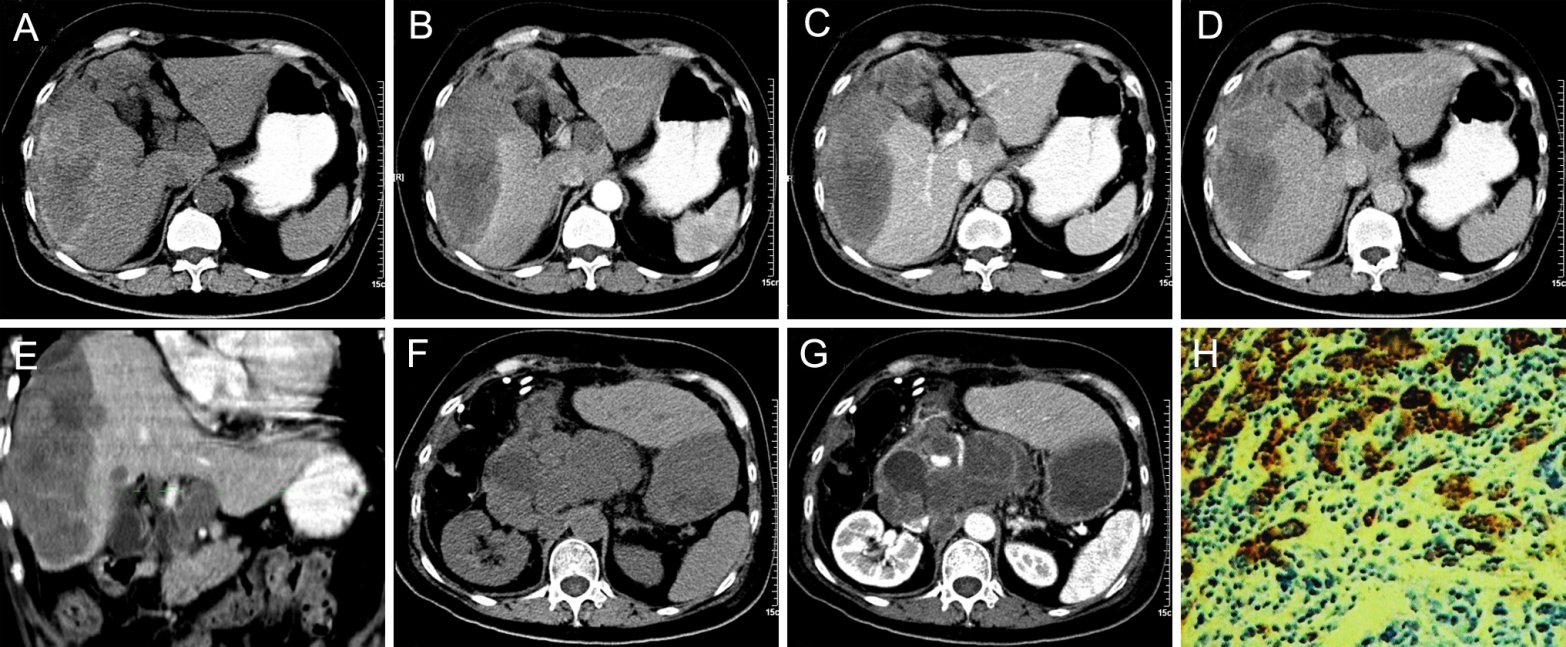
pPNET in a 66-year-old woman Precontrast CT images showed an irregular heterogeneous low-density mass with hemorrhage and ill-defined margins in the right lobe of the liver (A). The enhanced CT scan showed the mass had slight ring enhancement (B-D). Coronal reconstruction of the enhanced CT images showed the mass invaded the hepatic portal vein (E). The patient had recurrence and retroperitoneal lymph node metastases one month after surgery (F and G). The poorly differentiated small round tumor cells were CD99 positive (H × 100).
